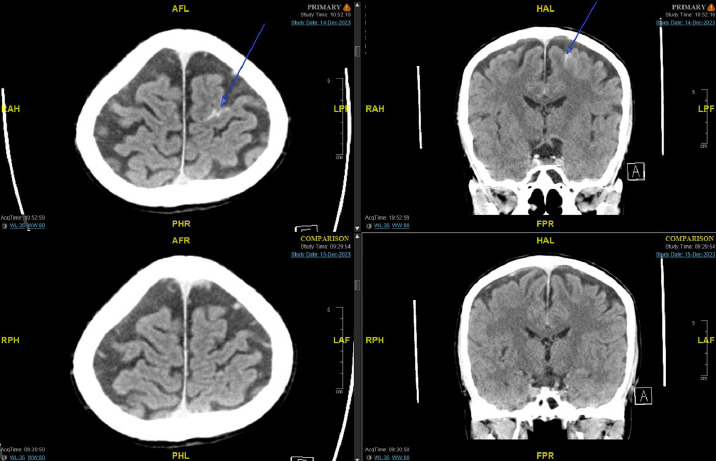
Top row - axial (left) cut of index brain CT; coronal (right) cut of index brain CT Arrow(s) highlighting acute subarachnoid haemorrhage (SAH) in the left precentral sulcus. Bottom row - axial (left) cut of interval brain CT conducted 22 hours after initial CT, coronal (right) cut of interval brain (CT. These images demonstrate the resolution of SAH.
